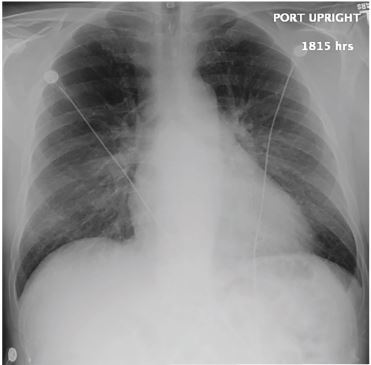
Anterior-posterior chest radiograph of a 55-year-old male with palpitations and dyspnea.
